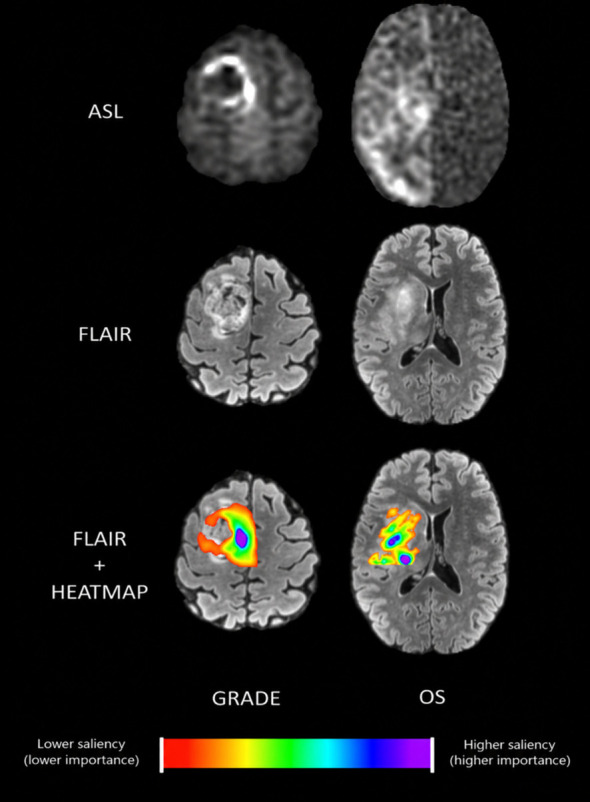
Model attribution maps for glioma grade and overall survival (OS) in two representative patients. For each patient, the same axial slice is shown across modalities. The first column shows ASL images, the second column shows native FLAIR images for anatomical reference, and the third column shows FLAIR images with integrated gradients (IG) saliency overlays. For grade prediction, attribution is predominantly localized within the enhancing tumor core, whereas for OS prediction, attribution extends into the peritumoral region, suggesting the model incorporates microenvironmental features relevant to prognosis. The color scale represents relative attribution intensity, with cooler colors (violet/blue) indicating a higher contribution to the model output.
